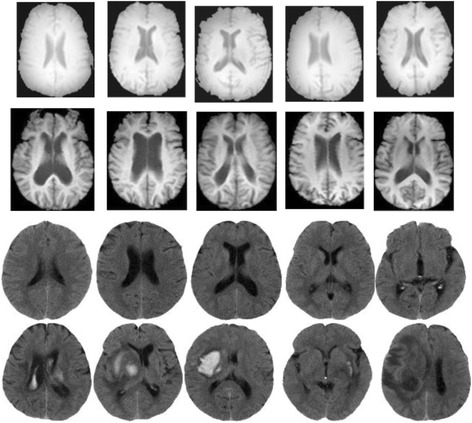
Examples of “Normal” and “Abnormal” images. Brain MRI images in the first row belong to “Normal” category and that in the second row are “Abnormal” ones. Brain CT images in the third row are “Normal” and that in the fourth row are “Abnormal”
